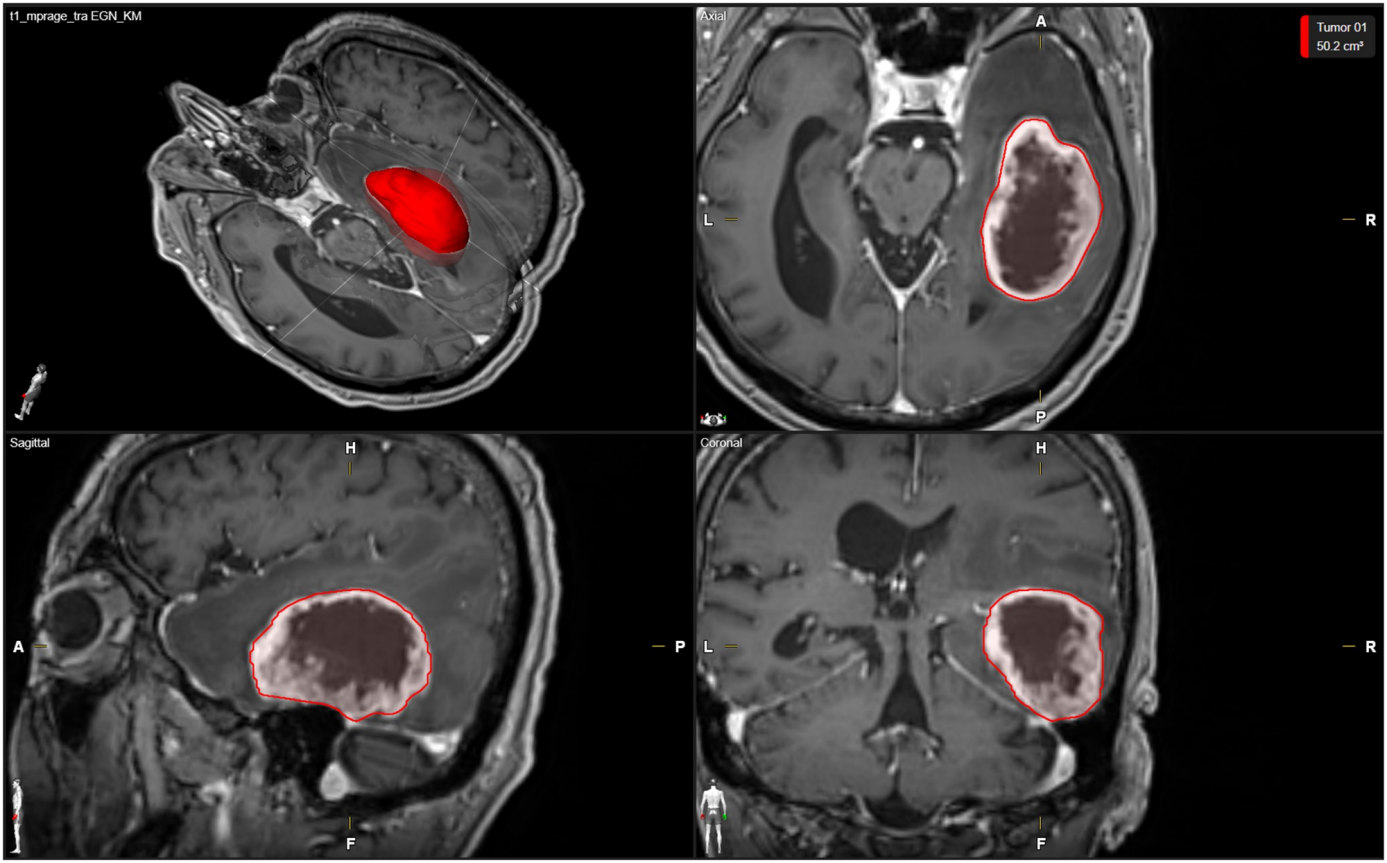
Example of volumetric analysis. The volumetric analysis of a right temporal HGG is shown in a 3-dimensional reconstruction, as well as in axial, sagittal and coronal planes. The tumor volume as determined based on contrast-enhanced T1-weighted images is highlighted in red.
